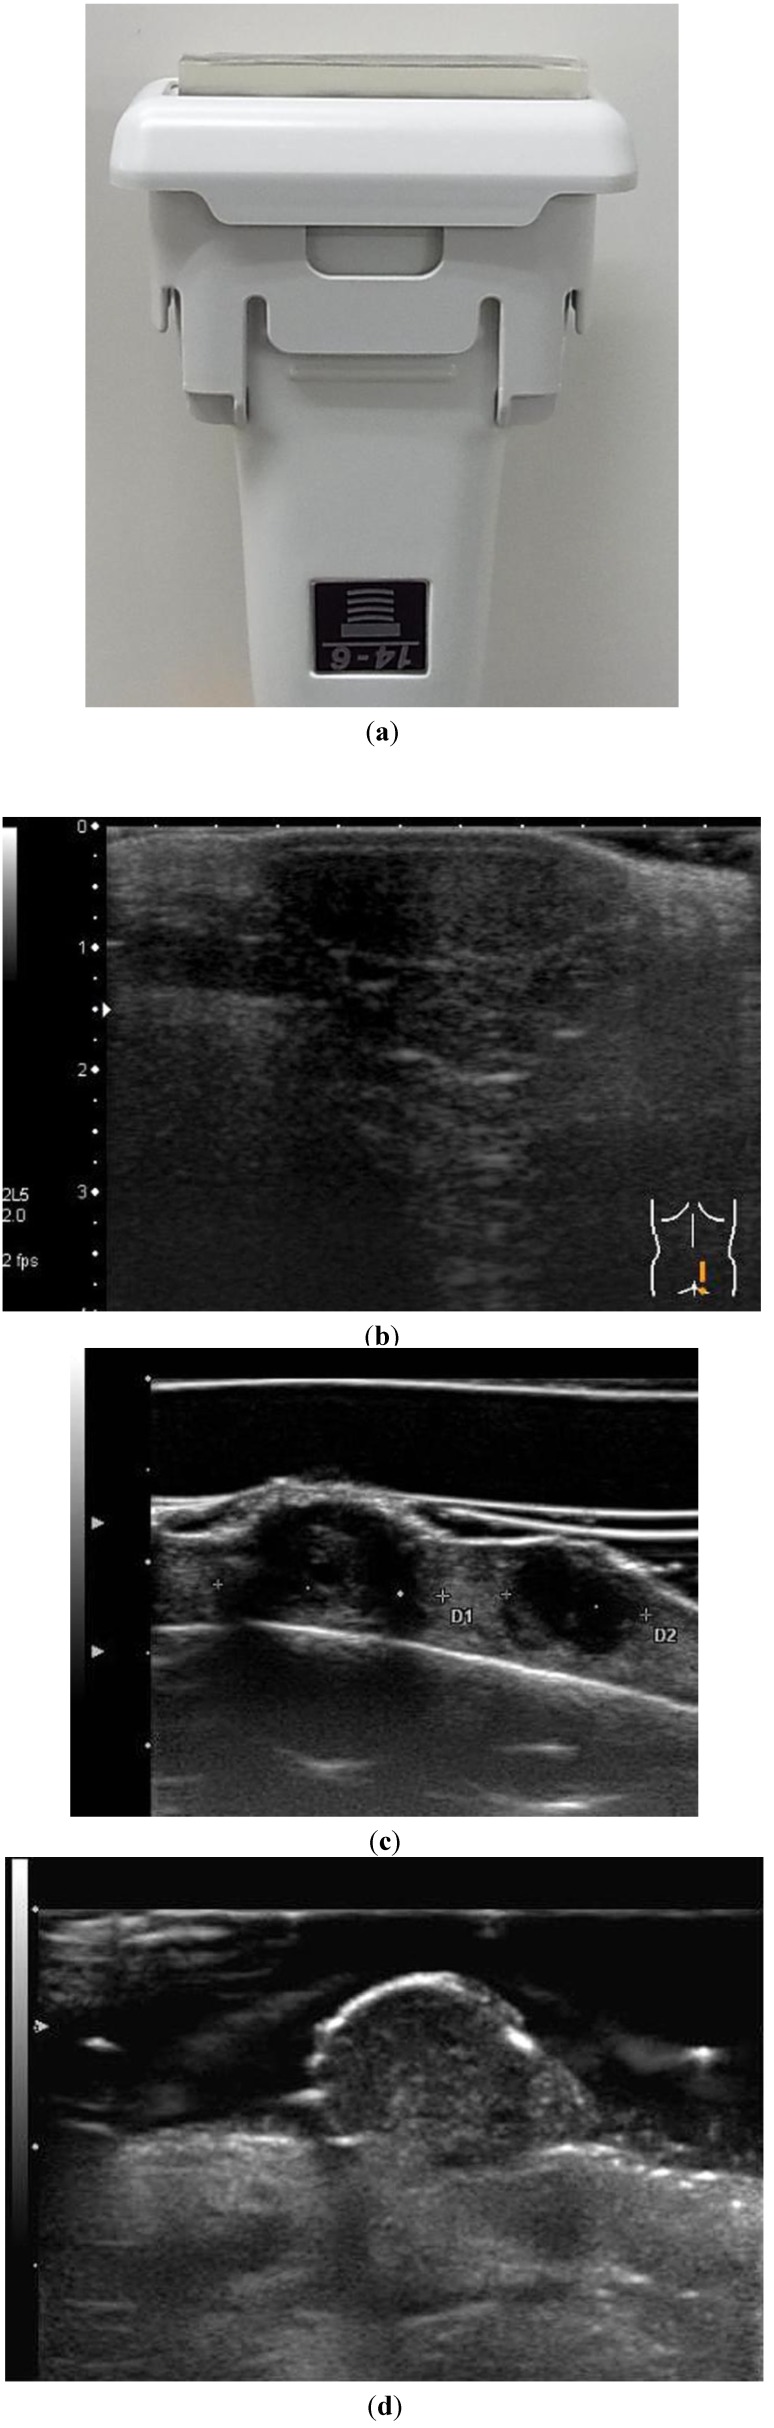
(a) Image shows an US probe and a gel stand-off pad. (b) A sonogram obtained with direct skin contact does not allow for optimal visualization of the cutaneous malignant melanoma. (c) A sonogram obtained with a gel stand-off pad allows for optimal visualization of the cutaneous malignant melanoma. (d) A sonogram obtained with a generous amount of US jelly similarly allows for optimal visualization of the cutaneous malignant melanoma.
